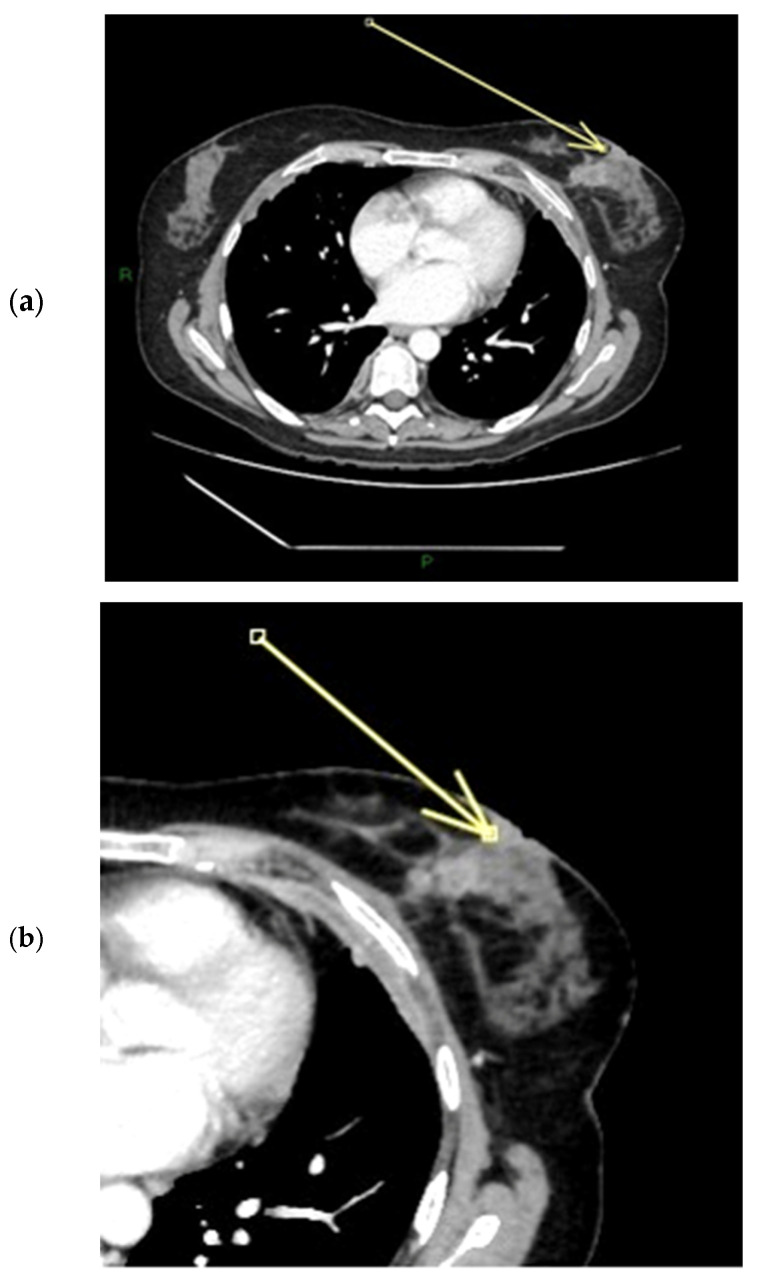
(a,b) Patient’s name D’A.L. In the ce-CT scan, the arrow shows enhancement of both the base and the body of the left nipple. Morphology is inverted, with skin thickening associated.
